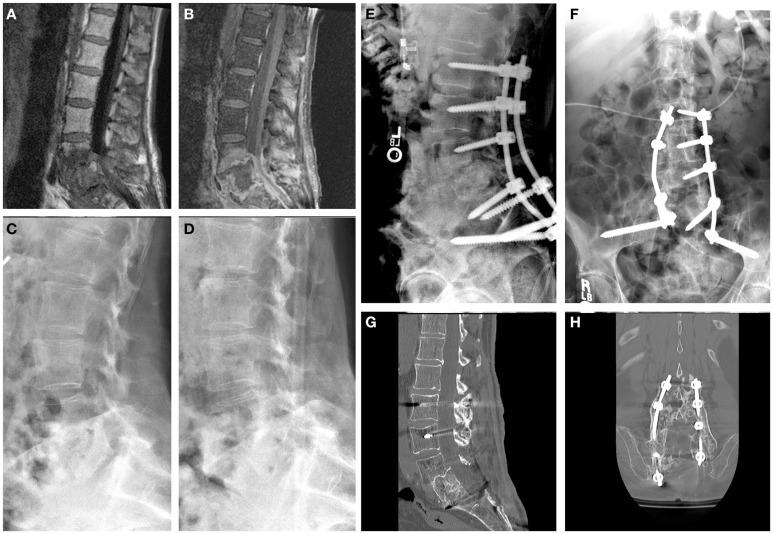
Patient 1. A 67-year-old woman with severe back pain presented after medical treatment of a polymicrobial sacral decubitus ulcer. Sagittal T1-weighted magnetic resonance images of the lumbar spine with (A) and without (B) contrast demonstrated PVDO at L4–L5–S1 with involvement of the vertebral bodies and endplates. L4–L5 spondylodiscitis with anterolisthesis was present as well as epidural and prevertebral abscesses. Preoperative upright radiographs before (C) and 1 month after (D) antibiotic therapy demonstrate progression from Grade I to Grade II L4–L5 anterolisthesis. Posterior-only decompression and fusion without formal debridement of the infected tissues was performed with pedicle screws at L2, L3, right L4, and S1 combined with S2AI screws (E,F); poor bone quality precluded instrumentation at L4 on the left and at L5. At 2 years, sagittal (G) and coronal (H) CT reconstructions demonstrate stable fusion at the lumbosacral junction.
